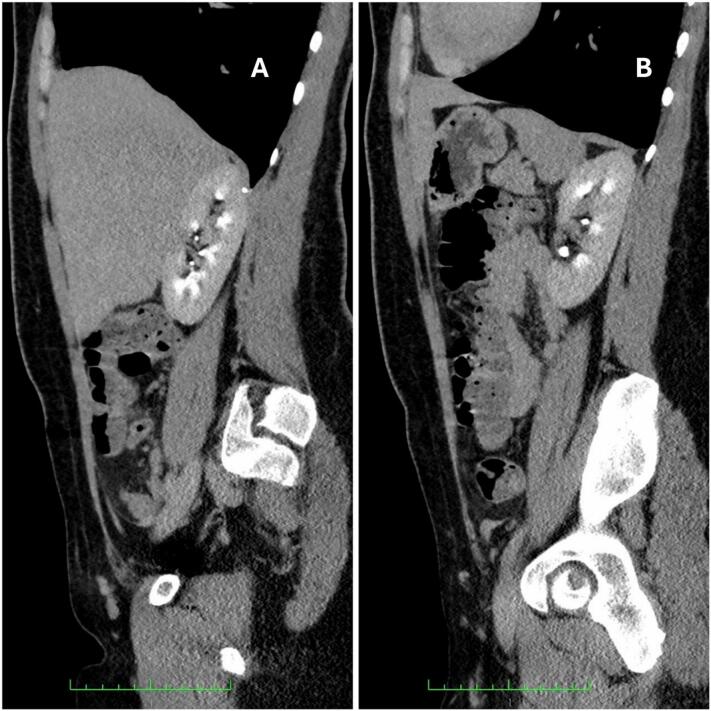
Abdominopelvic CT scan, sagittal views. (A) Shows the patient's right side of the body without any enhancing renal lesions. (B) Is only remarkable for an enlarged, nonspecific, para-aortic lymph node in the patient's left side of the body. This left para-aortic lymph node enlargement remains under close observation, with follow-up imaging planned to monitor any changes in size or characteristics.
